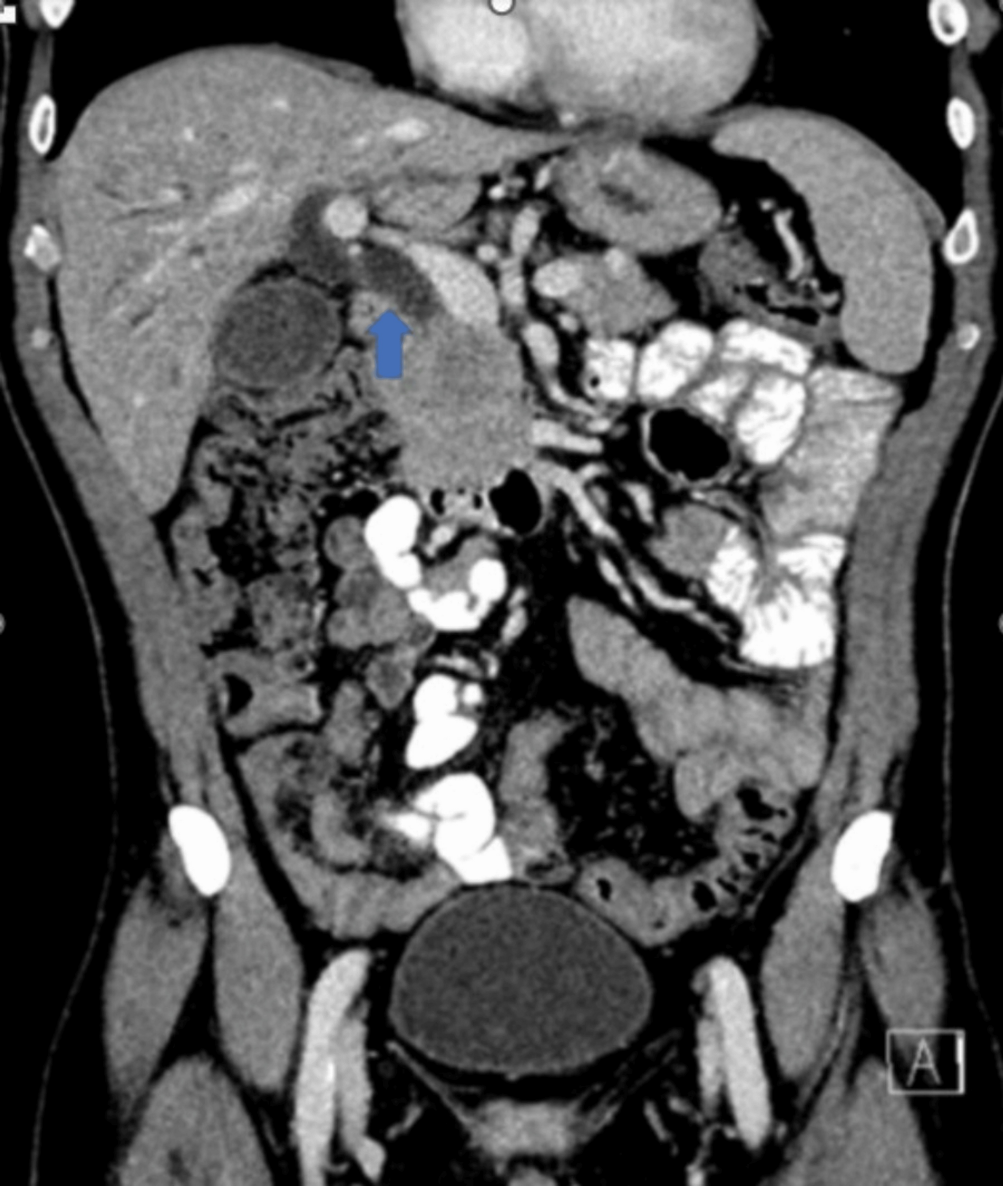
Coronal contrast-enhanced CT image demonstrating marked common bile ductal dilatation of 13 mm (blue arrow). Significant intrahepatic biliary ductal dilatation (not seen on this image) was also noted.
